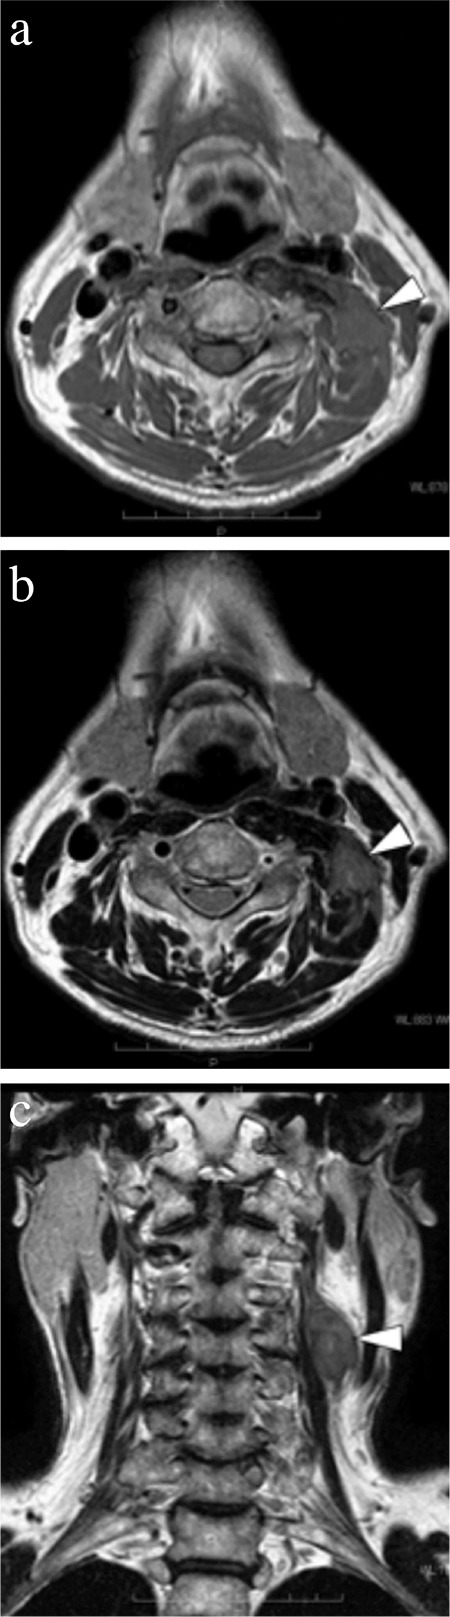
Magnetic resonance imaging of left posterior neck mass (white arrow head). Axial view of T1-weighted (a) and T2-weighted (b), and coronal view of T2-weighted (c).
